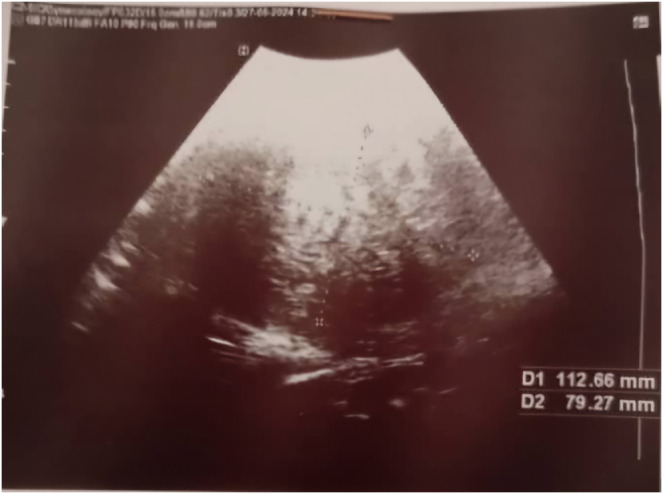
Transvaginal pelvic ultrasound showing a markedly enlarged uterus with a well-circumscribed, heterogeneous, hypoechoic mass located on the right uterine side, measuring approximately 8 × 11 cm. The endometrial stripe is displaced but not invaded. The mass was initially interpreted as a large intramural leiomyoma. Both ovaries appear normal in size and echotexture, with no evidence of masses or calcifications.
